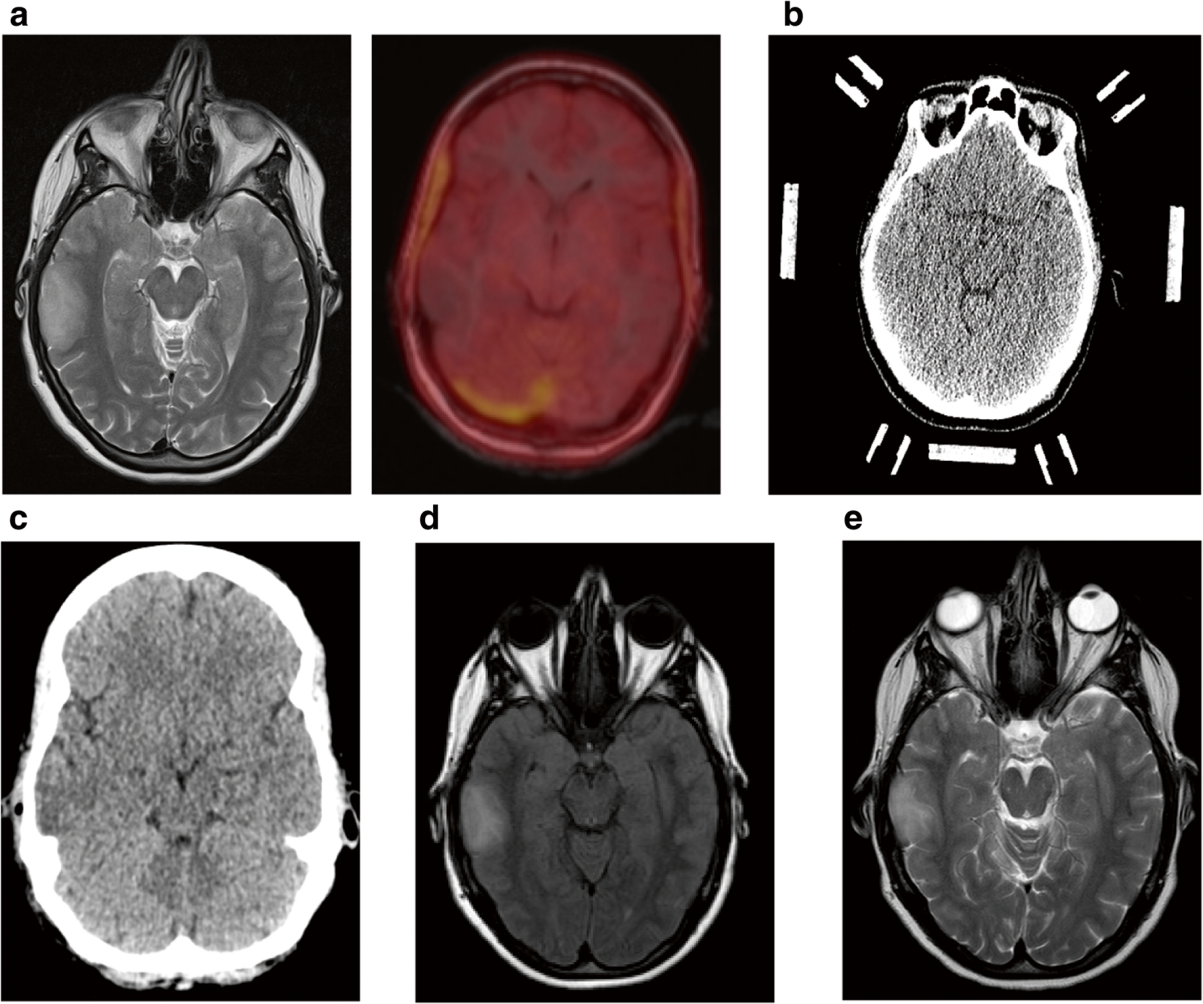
Patient was admitted for biopsy with a T2 diffuse hyperintense cortico-subcortical lesion within the right temporal lobe (a, left panel) showing no tracer enhancement in FET PET MRI (a, right panel) and no MRI contrast enhancement (not shown). Biopsy was planned with CT in a stereotactic frame (b). Postbiopsy CT showed no biopsy-associated complications (c). Since biopsy was non-diagnostic, follow-up was performed after 3 weeks (d) and 7 months (e) showing no change in MRI findings
